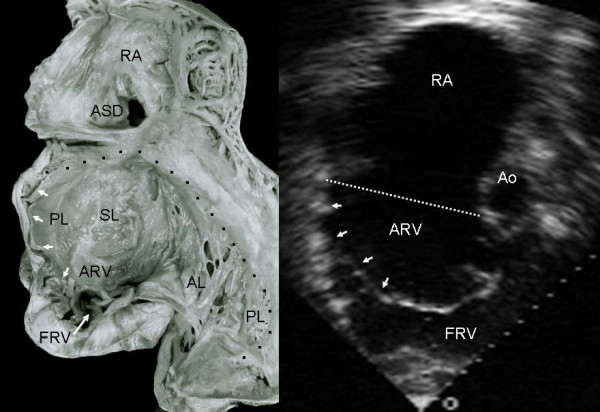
Internal view of the right chambers shows extreme tethering of the tricuspid septal and posterior leaflets to the ventricular wall (short arrows) and severe reduction of the functional portion of the right ventricle (long arrow). Note the atrial septal defect. The echocardiographic image shows the hammock-like aspect of the tethered posterior and septal leaflets that separate the atrialized and functional portions of the right ventricle. The atrialized portion of the right ventricle predominates. Ao: Aorta. Other abbreviations as before.
